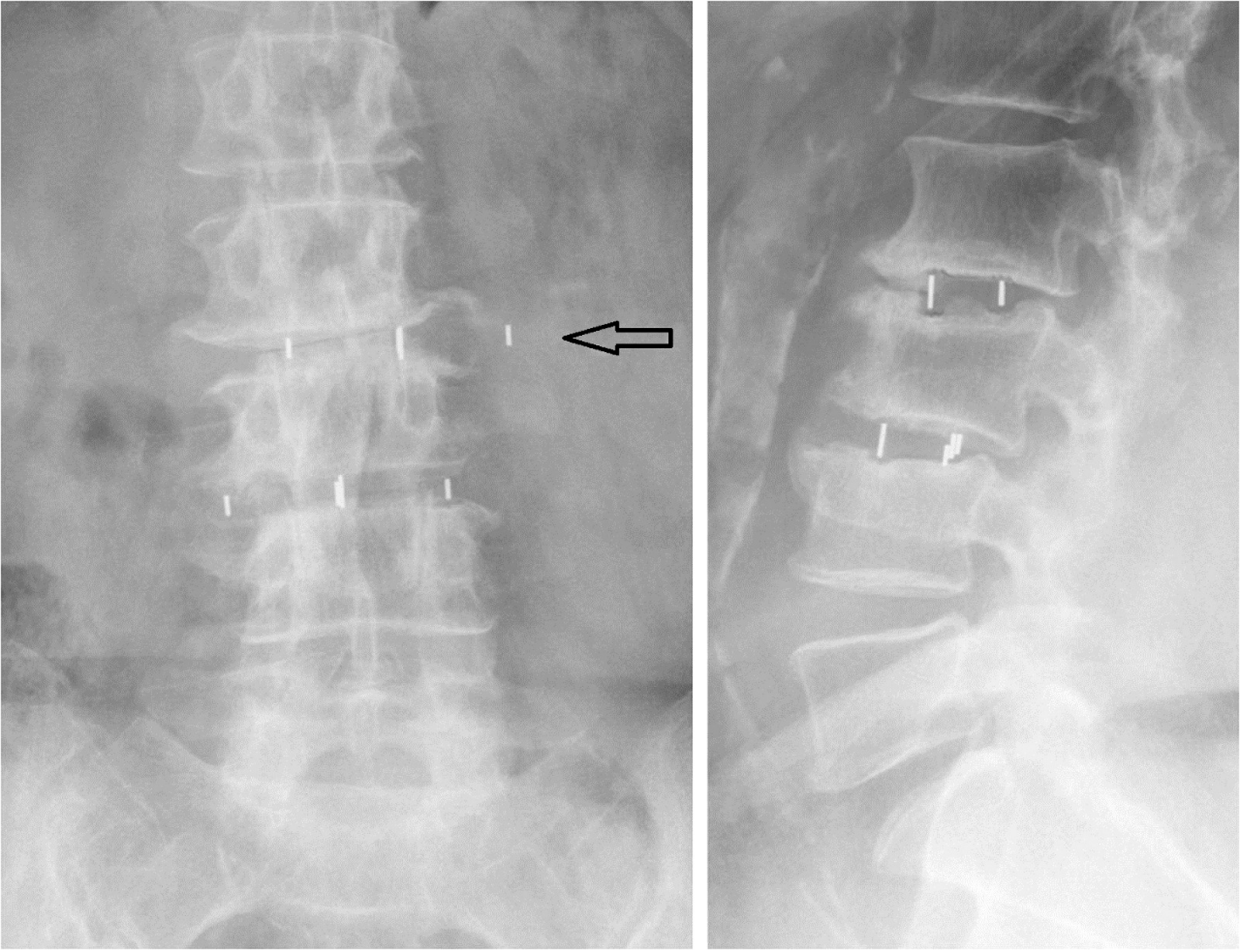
Three month postoperative x-ray Lateral cage migration at L2-3
